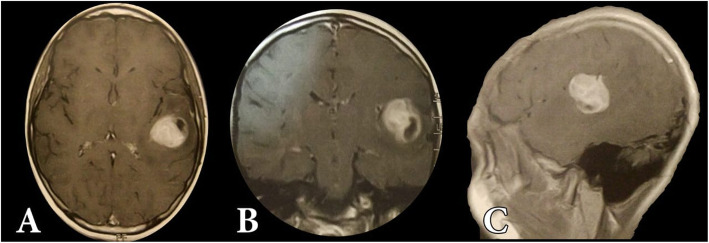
Preoperative axial (A), coronal (B), and sagittal (C) MRI with gadolinium images of a 14-year-old female patient presented with seizures, showing lt sided sol related to Wernick’s area. She was operated under local anesthesia and went home 2 days after surgery
